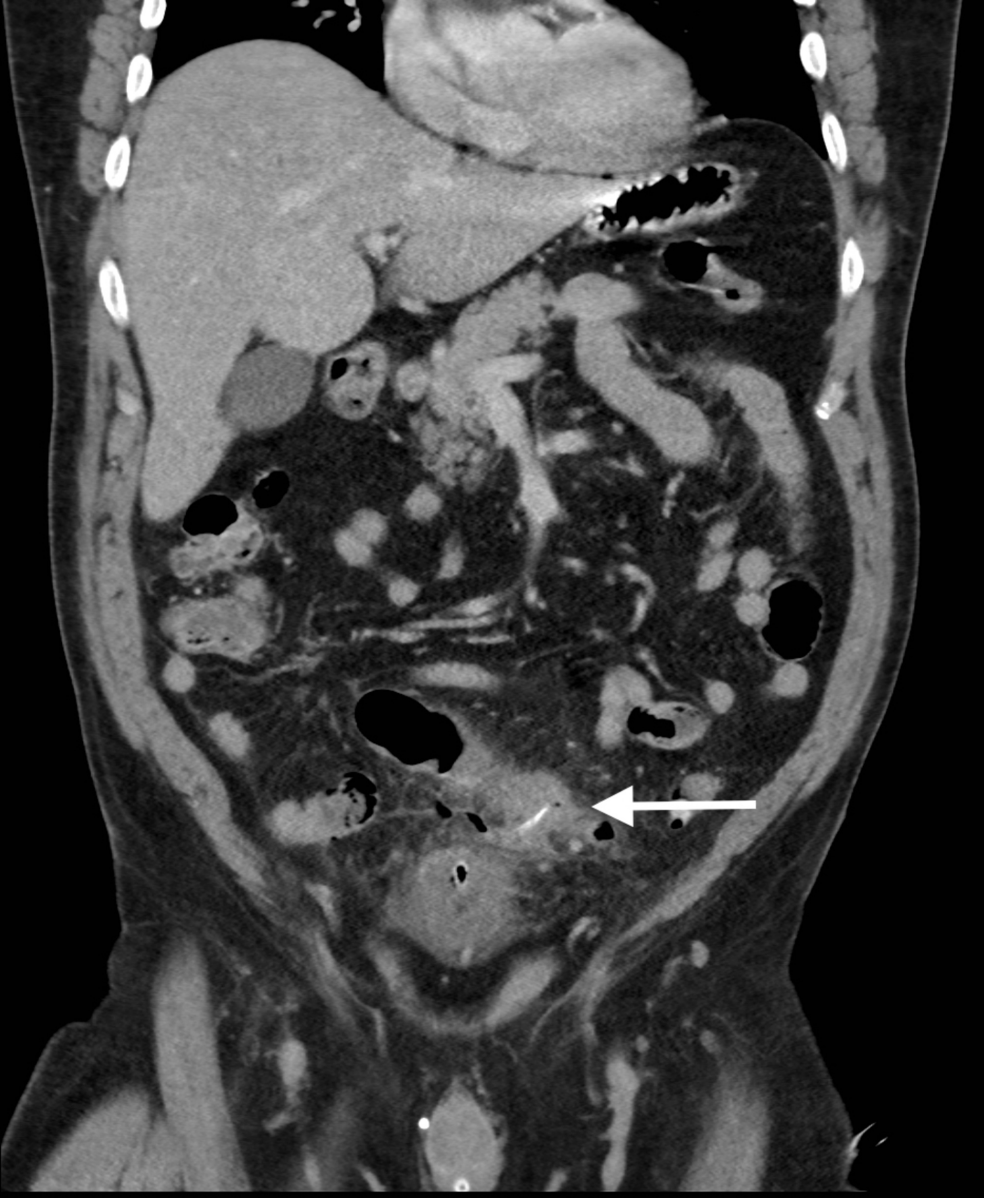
Coronal view of an abdomino-pelvic computed tomography scan. Linear hyperdensity is visible within the inflamed sigmoid colon (white arrow).
